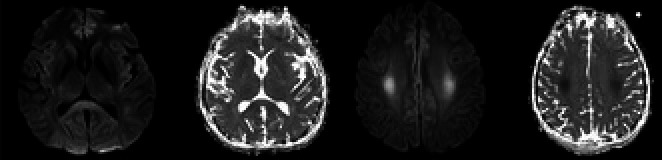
FIGURE 2 Follow‐up brain MRI performed 4 days later demonstrating regression of the lesions observed on the initial MRI.
